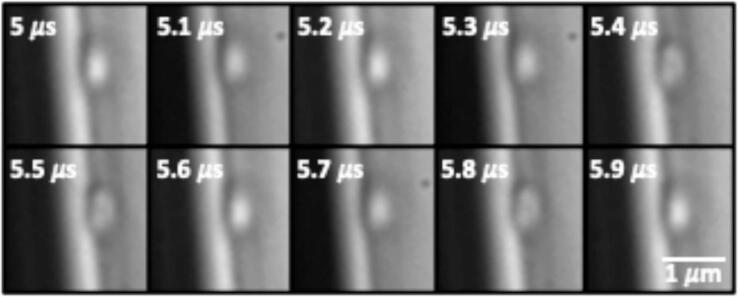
Images of 1.2 µm diameter crevice bubble driven by 5.0 MHz Doppler pulses. Frames were captured at 200 fps with the respective time delays. There was no noticeable change of the bubble radius, which was consistent for all tested parameters.
